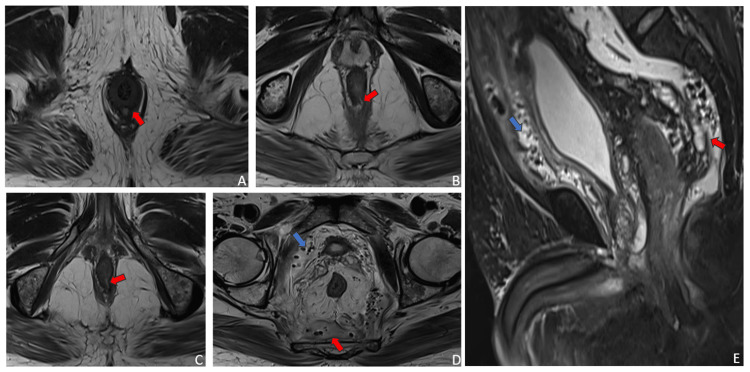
(A) MRI axial T2WI showing the external opening of the fistula at the 6 o’clock position appearing hyperintense on T2. (B, C) MRI axial T2WI showing the ramifications of the fistulous tract opening into the presacral region. (D) MRI axial T2WI showing heterogeneously hyperintense collections with hypointense foci of air in the presacral region (red arrow) and perivesical region anteriorly (blue arrow). (E) MRI STIR sagittal image showing walled-off presacral collection with air foci extending superiorly into the retroperitoneum and anteriorly in the perivesical region (red arrow). MRI: magnetic resonance imaging; T2WI: T2-weighted image; STIR: short tau inversion recovery
